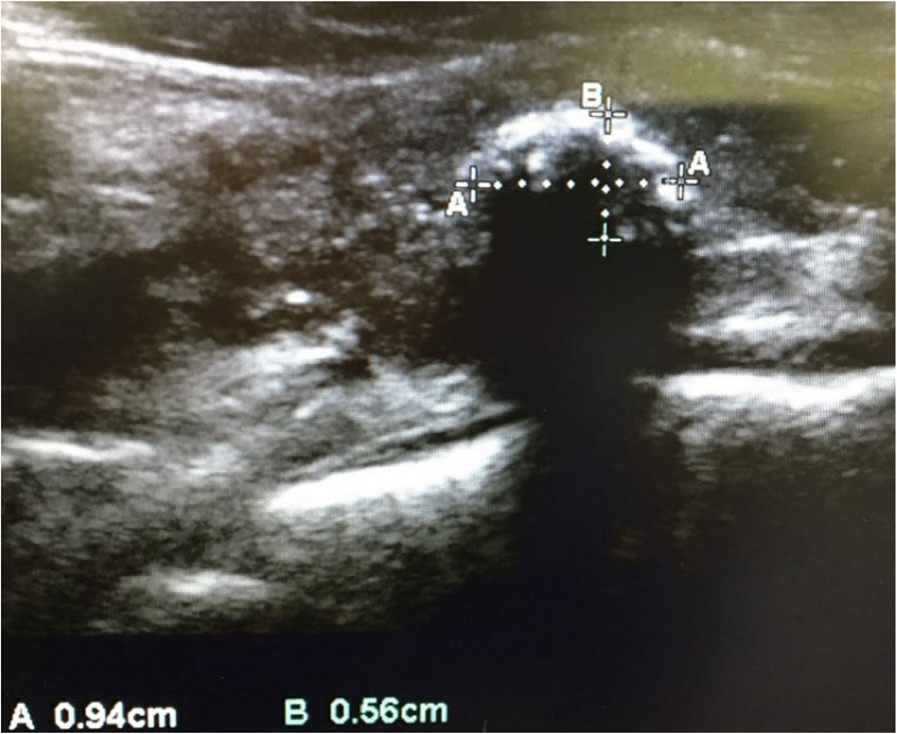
Ultrasonography of the thyroid. A mass with a calcification and an acoustic shadow is observed in the thyroid
